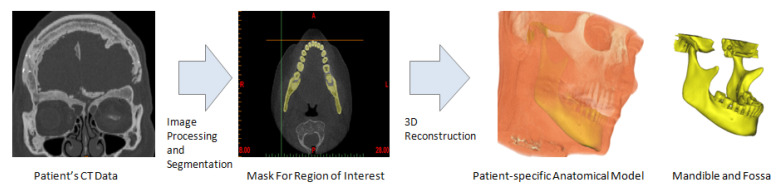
Subject-specific 3D anatomical reconstruction of the patient’s mandible and fossa eminence performed from computed tomography (CT) data using Mimics software.
